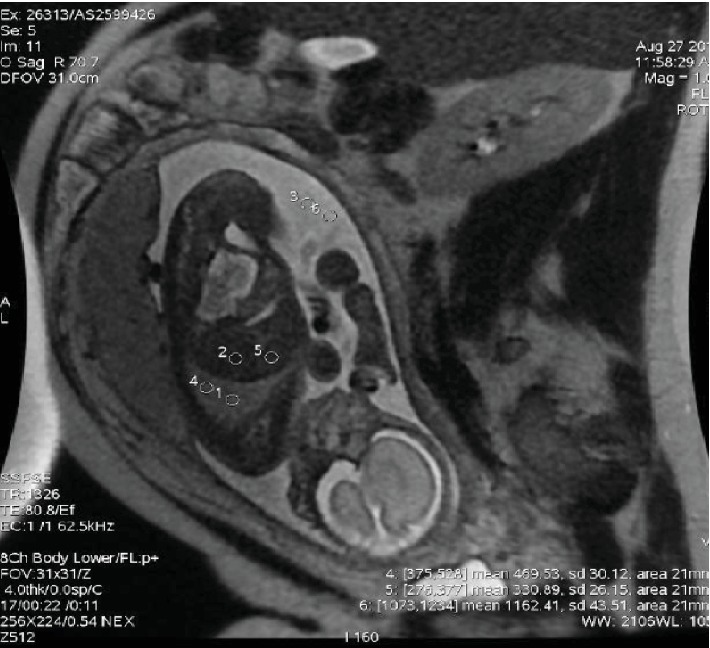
Coronal SSFSE T2-weighted image where 6 ROI (regions of interest were placed: 2 on the lung, 2 on the liver, and 2 on the amniotic fluid).
